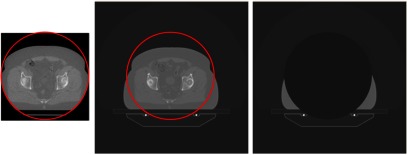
Example of Truncation correction for Prostate case. From left to right: MVCT image showing TomoTherapy scanning circle, planning CT showing TomoTherapy scanning circle, and finally, the mask used for this slice of the patient CT—figure reproduced from Thomas *et al* (2016) © 2016 The Authors. Published by the British Institute of Radiology. CC BY 4.0.
